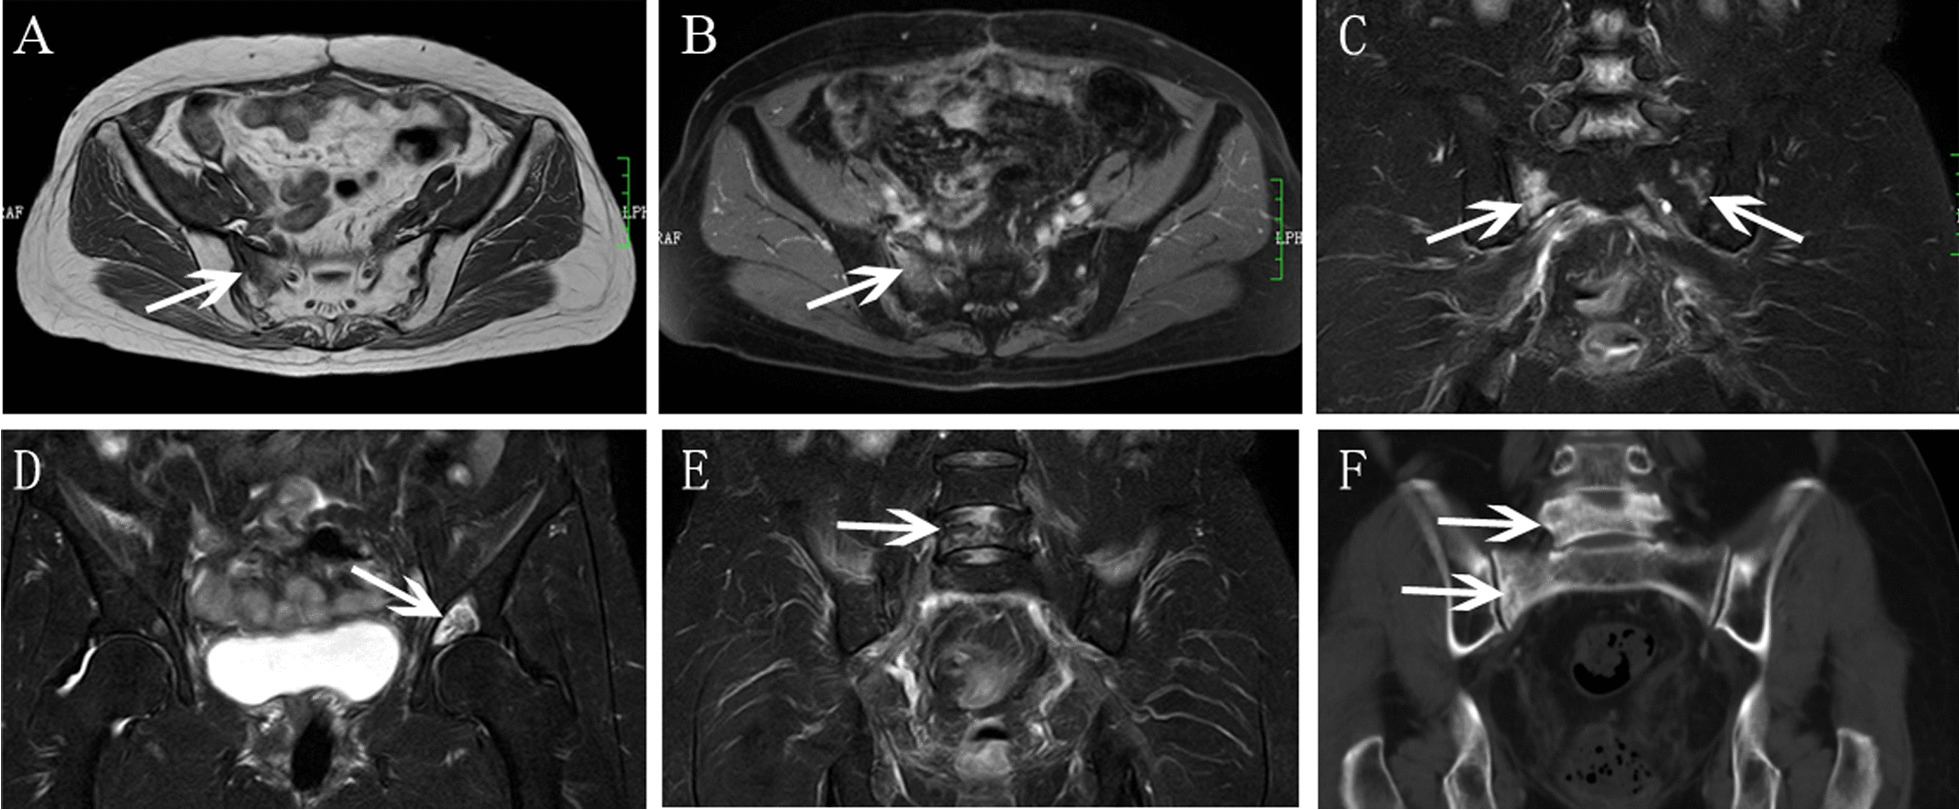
Bilateral SIFs had coexistent L5, acetabulum fractures in a 68-year-old woman with cervical cancer after radiotherapy. A, B Axial TWI and enhanced T1WI showed right sacrum abnormal signal (arrow). C Coronal FS-T2WI showed abnormal signal in bilateral sacrum, right sacrum showed severe bone marrow edema, and left sacrum showed mild bone marrow edema (arrow). D, E Coronal FS-T2WI showed acetabulum and L5 fractures. F Coronal CT image showed osteosclerosis change in L5 and right sacrum
